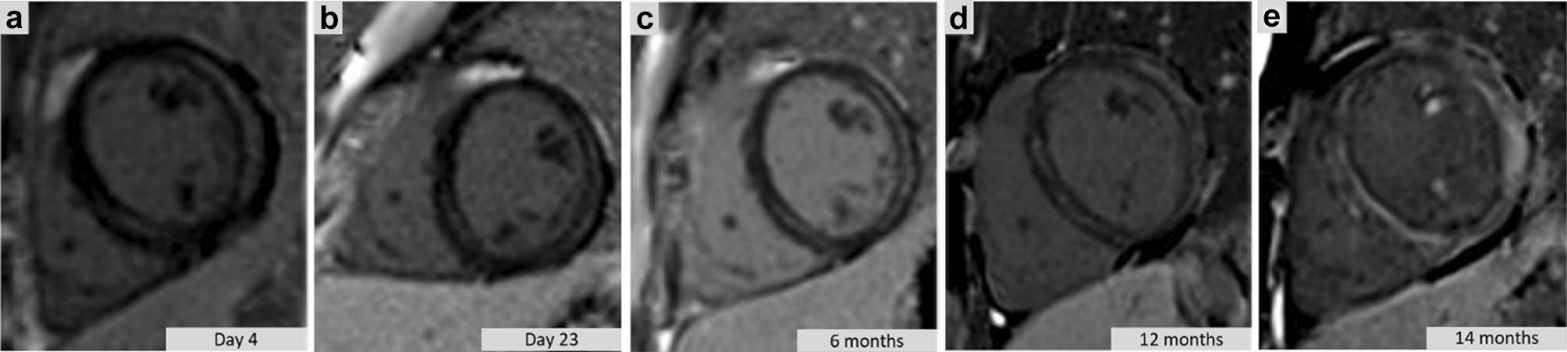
a–e Serial cardiac magnetic resonance images of patient 1 at different times after the first cardiac arrest revealing subepicardial late gadolinium enhancement (details in text)
